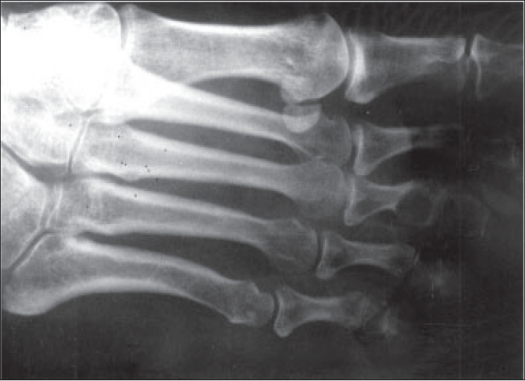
X-ray of the same foot after 18 months showing lytic lesion of the proximal phalanx of third toe
